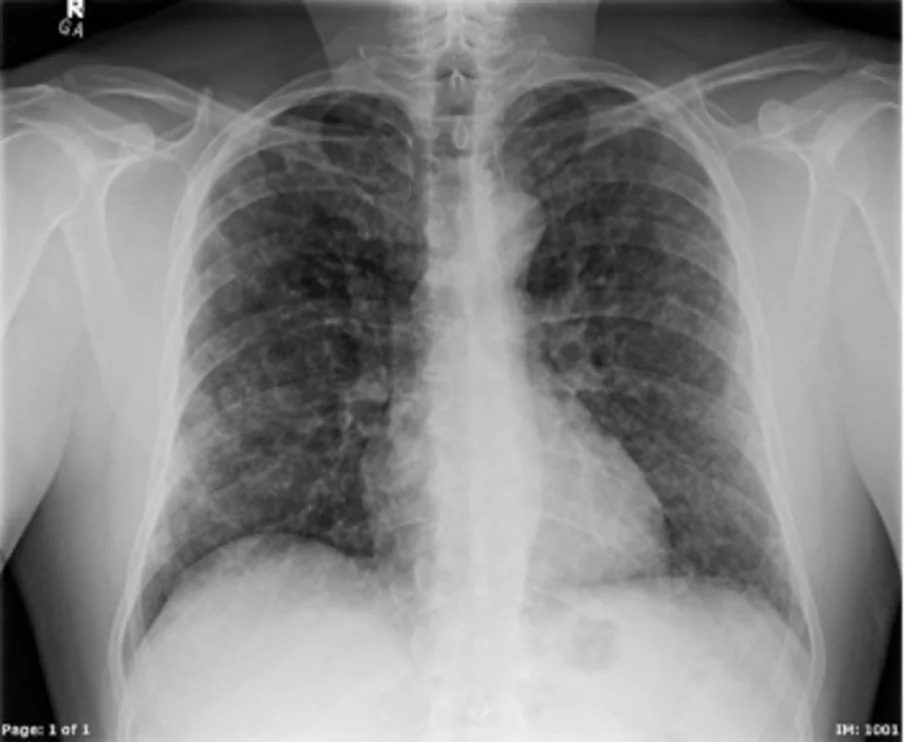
Upright posteroanterior chest radiograph demonstrated diffuse nodular opacities.
